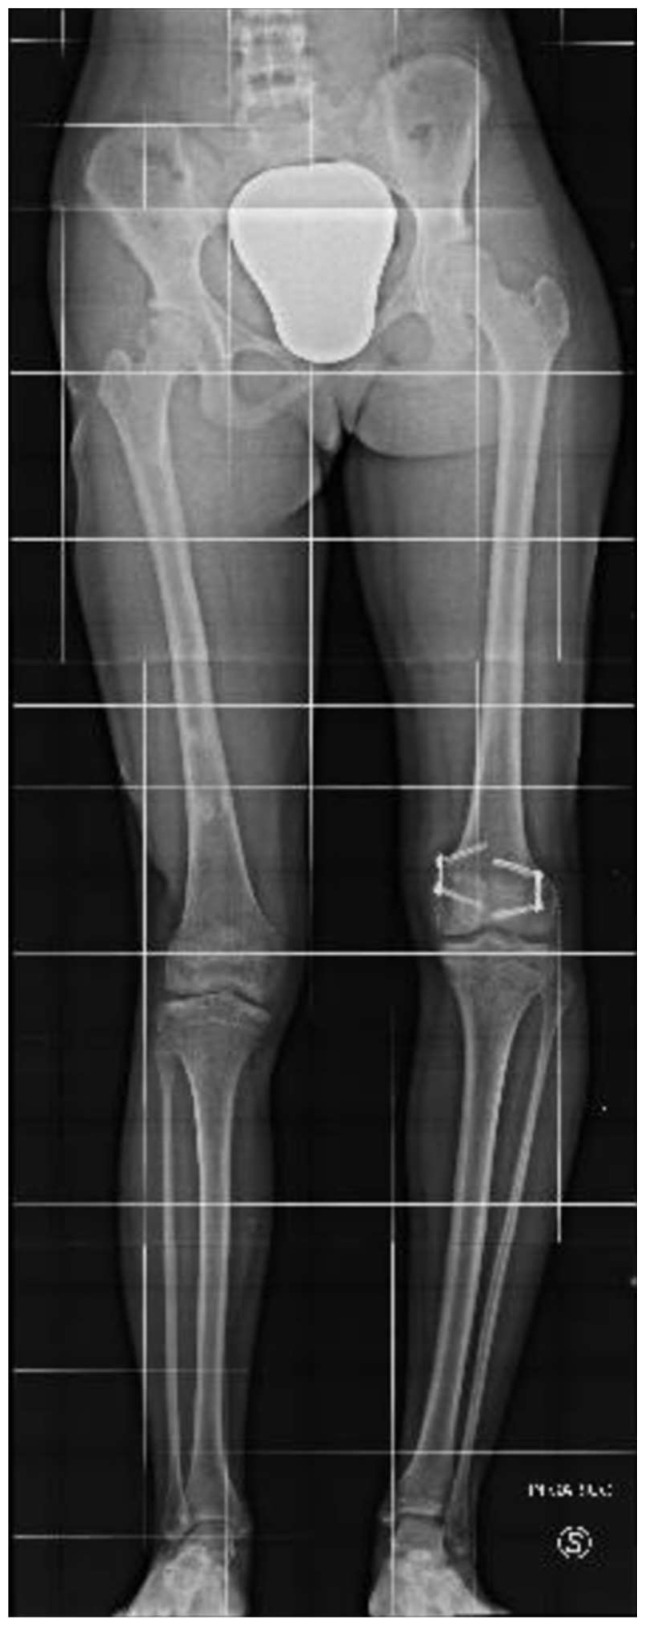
Radiographs showing residual tibial hypoplasia (≅40 mm) and valgus knee deformity.
